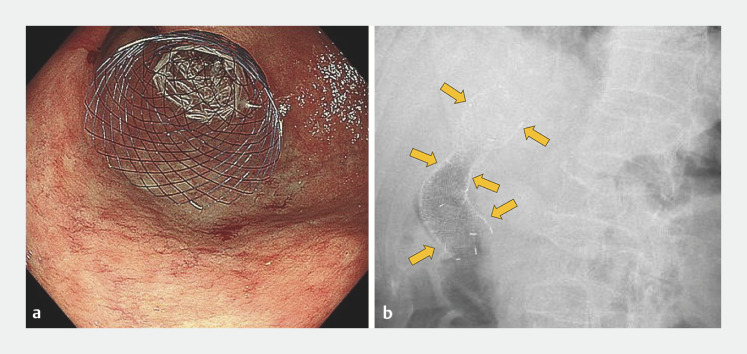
A duodenal covered self-expandable metal stent placed with the uncovered proximal flare remaining in the pyloric canal is seen on: a endoscopic view; b radiographic imaging.
